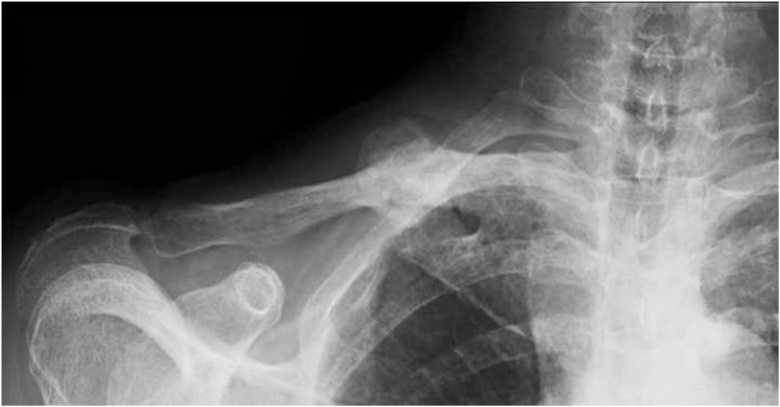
Anteroposterior X-ray image of the clavicle taken 10 months after the first admission.
